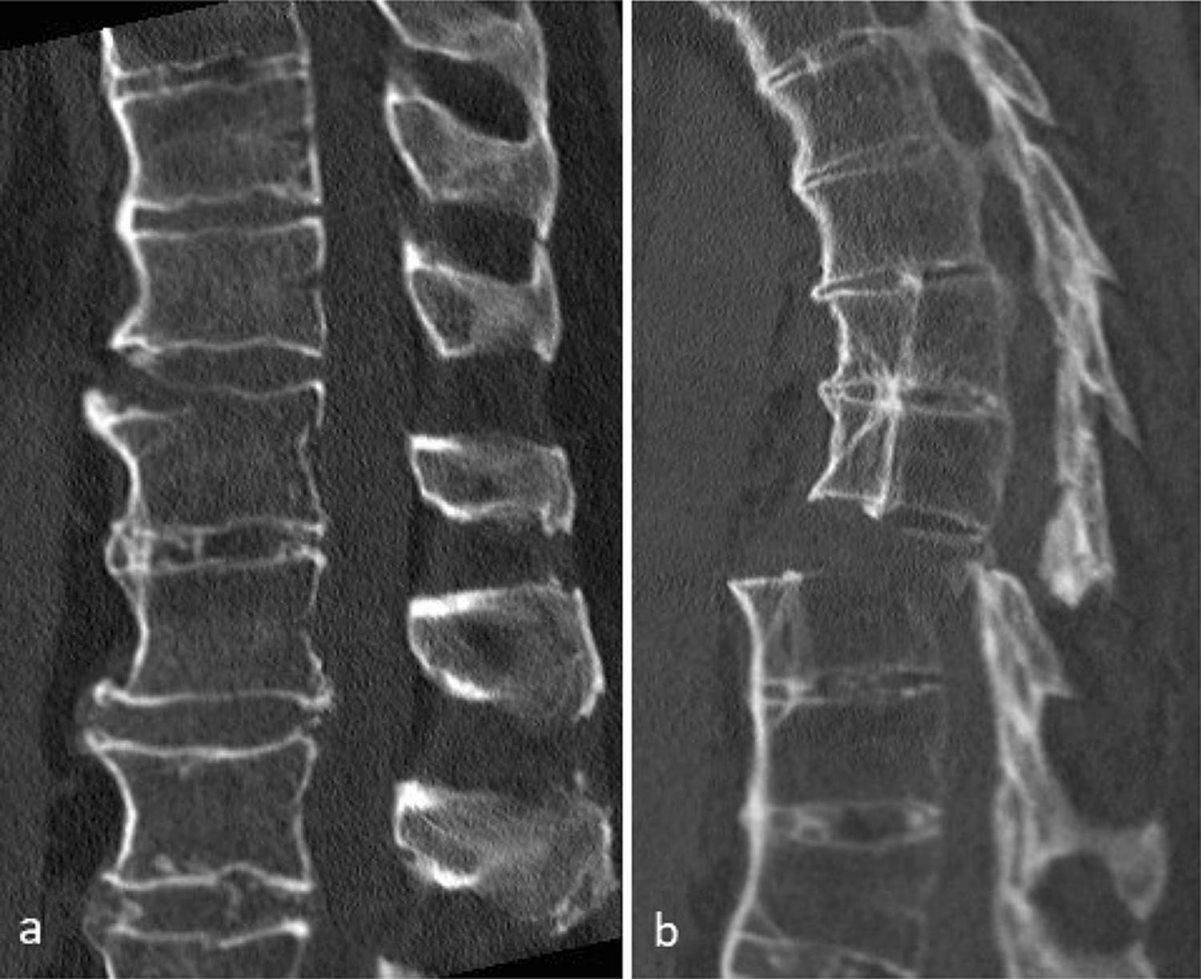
Typical examples of sagittal CT images of a distracted fracture. a B3-Type according to AOSpine. b C-Type according to AOSpine
